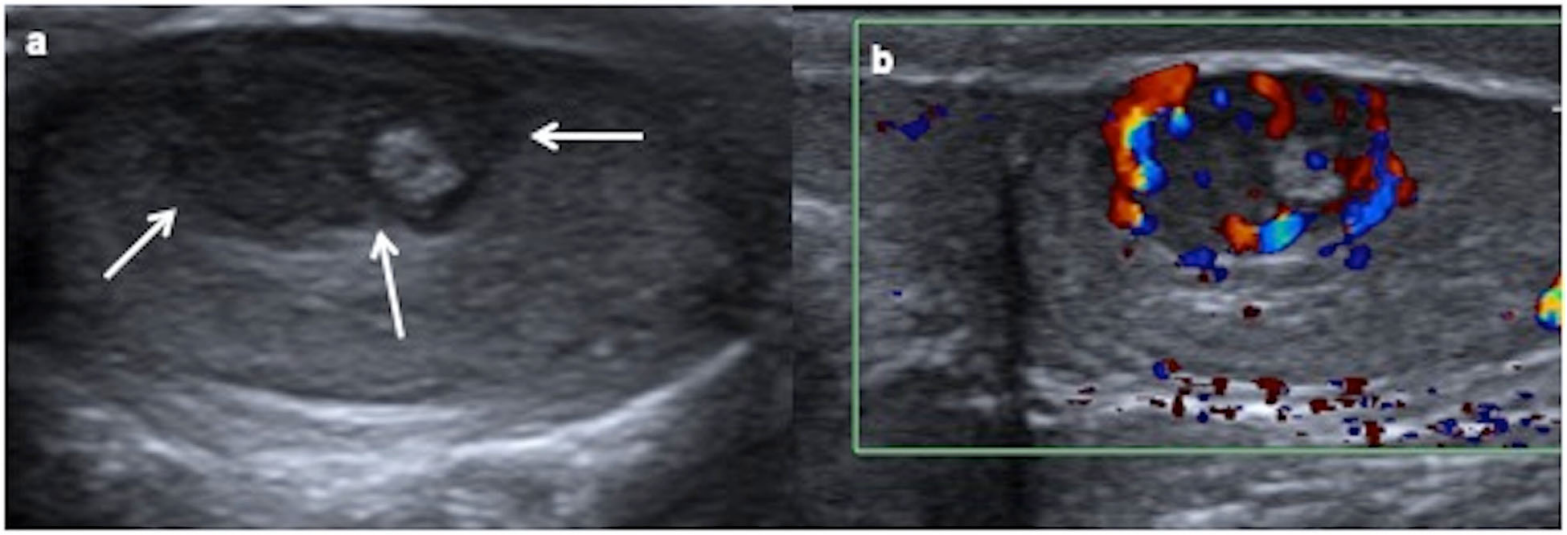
Leydig cell tumour. A 7-year-old boy presents precocious puberty with a high level of testosterone. Scrotal ultrasound is performed. a Longitudinal view of the left testis shows a well-delimited lobulated heterogeneous mass (arrows) and (b) colour Doppler image shows peripheral and central vascularization of the mass. Intraoperative biopsy diagnoses a Leydig cell tumour and a testis sparing surgery is carried out
